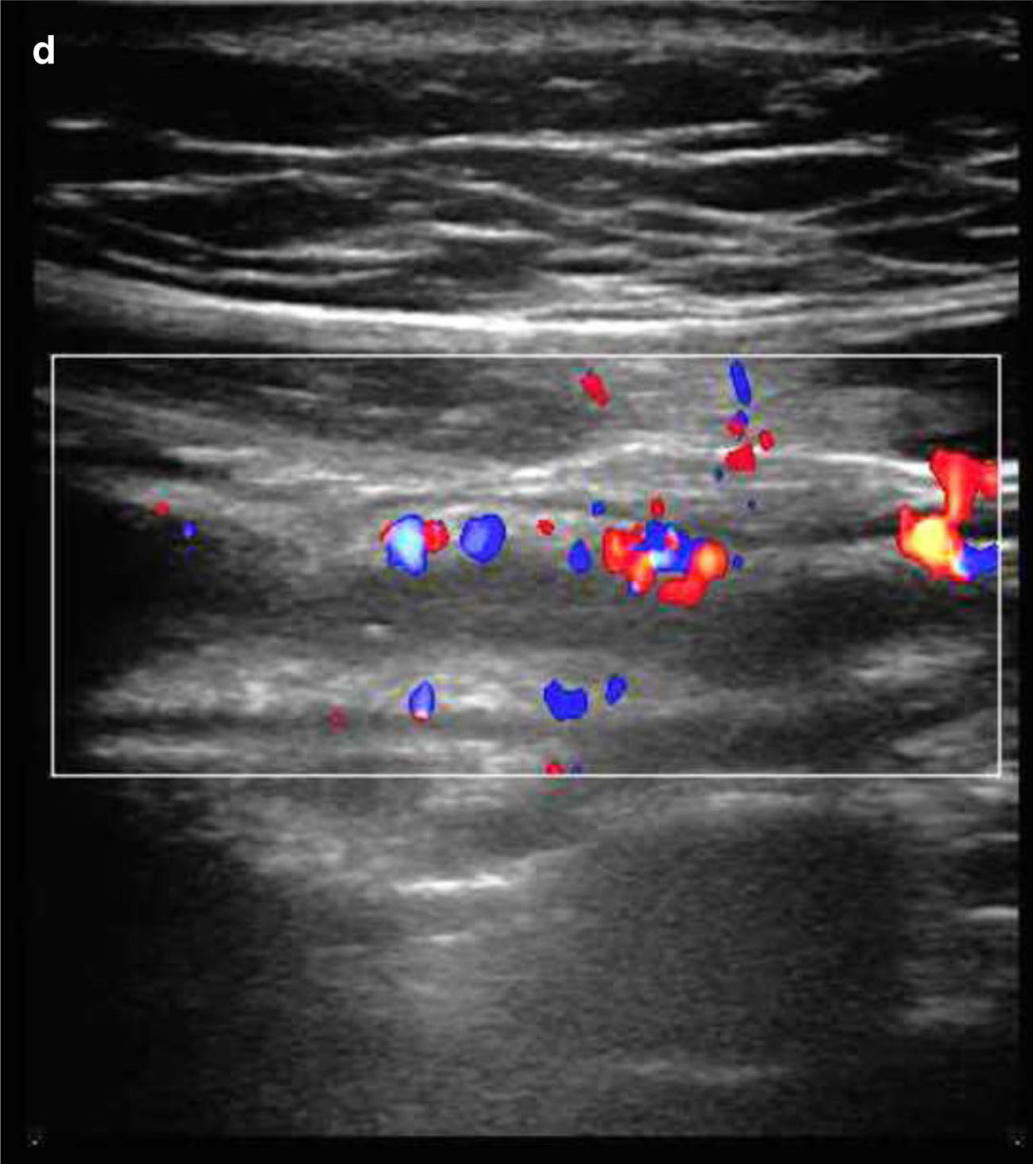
Sagittal greyscale US (a) in a 13-year-old female: ileocolic Crohn’s disease. Bowel wall thickening with modification of normal stratification. Sagittal colour Doppler (b), axial power Doppler (c) plane: active Crohn’s disease. Increased vascularity in the thickened bowel wall segments. Sagittal colour Doppler image (d) of the left colon in a 15-year-old male with UC shows thickened and moderately hyperaemic bowel wall segment with preservation of normal stratification
